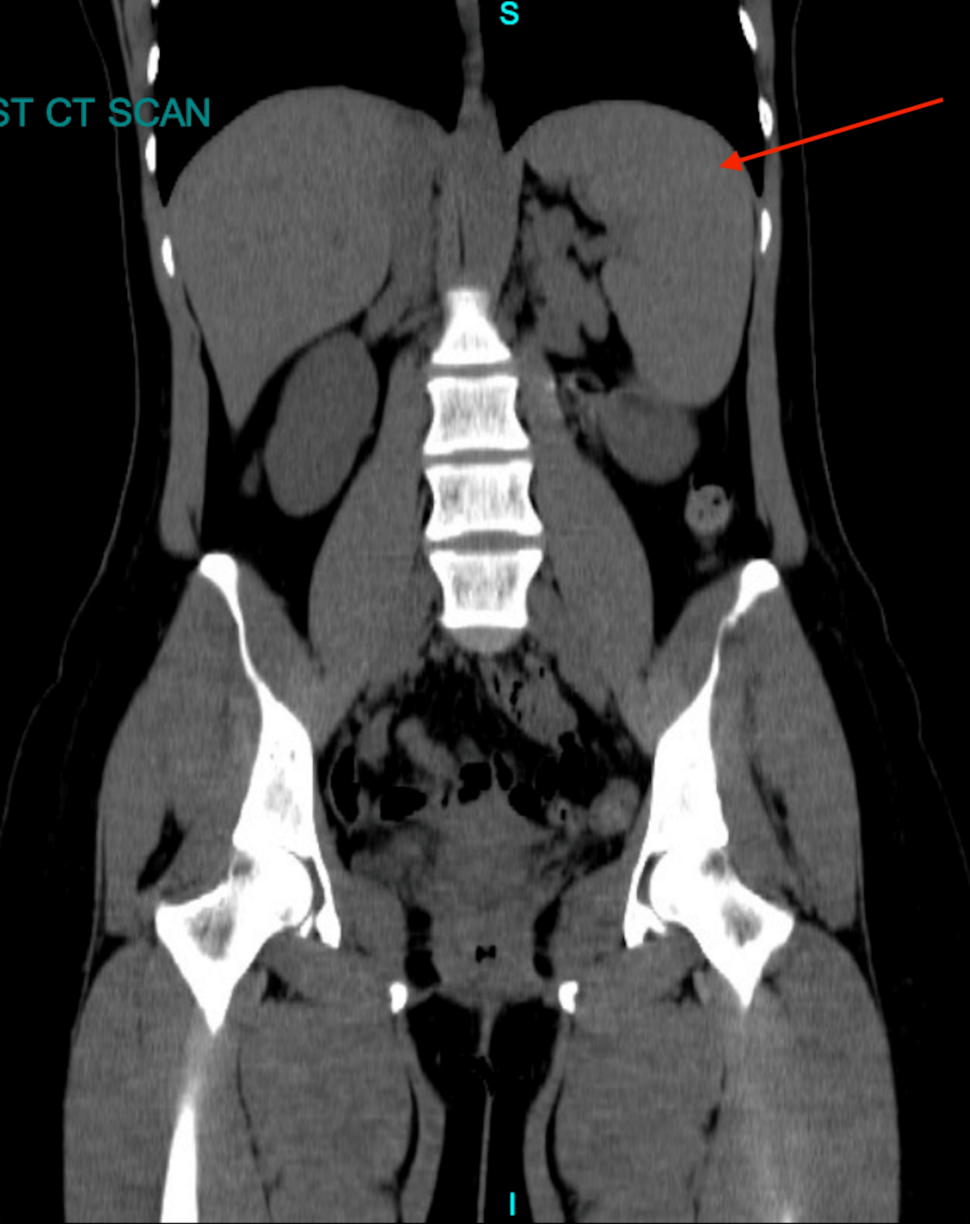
Coronal view of the abdomen CT scan The red arrow is pointing at the spleen, which shows splenomegaly.
